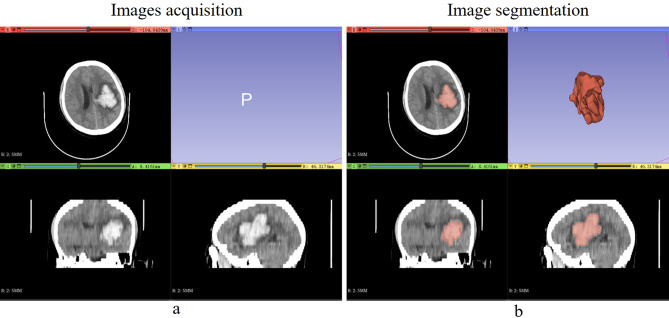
Noncontrast CT images was semi-automatic segmentation. *CT* computed tomography.
